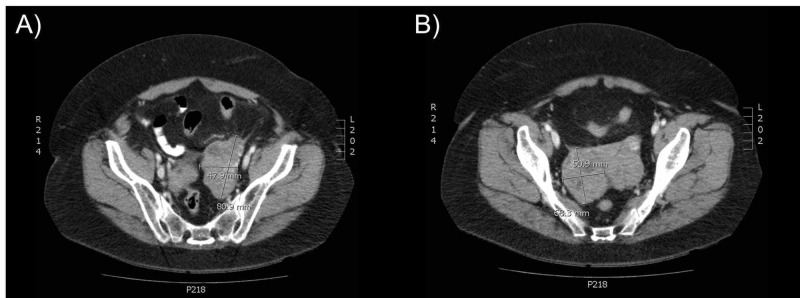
CT images of ovarian masses found five years after initial diagnosis and treatment of lung LCNEC. The patient presented with bilateral ovarian masses and neurological symptoms during follow-up surveillance. Images reveal bilateral ovarian masses: (A) Left: 8.1 x 4.8 cm; (B) Right: 6.8 x 5.1 cm. Surgical resection confirmed these masses as LCNEC of pulmonary origin. LCNEC: Large cell neuroendocrine carcinoma
